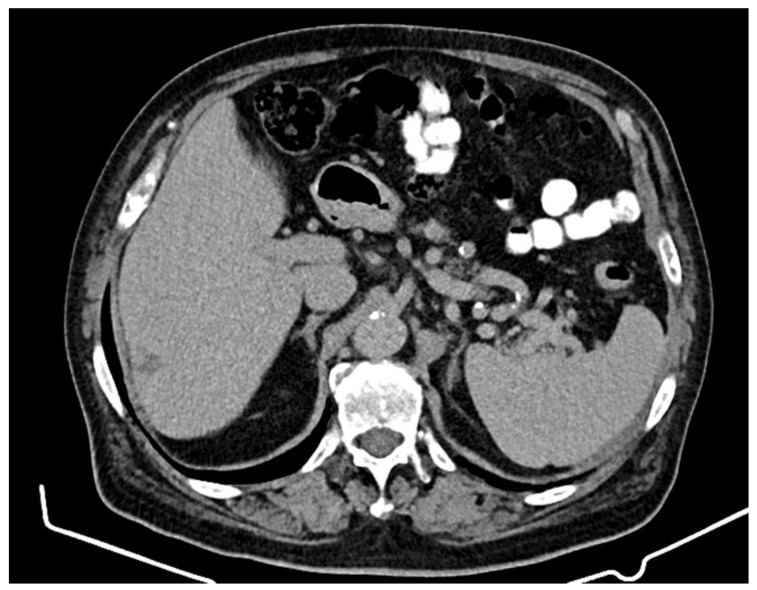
Computed tomography (April 2021): liver with non-homogenous structure, with the presence of a hypocapturing mass with axial diameters of 28/26 mm. Middle and lower interaorticocaval and lumboaortic retroperitoneal lymph nodes with maximal axial diameters of 17/11 mm.
